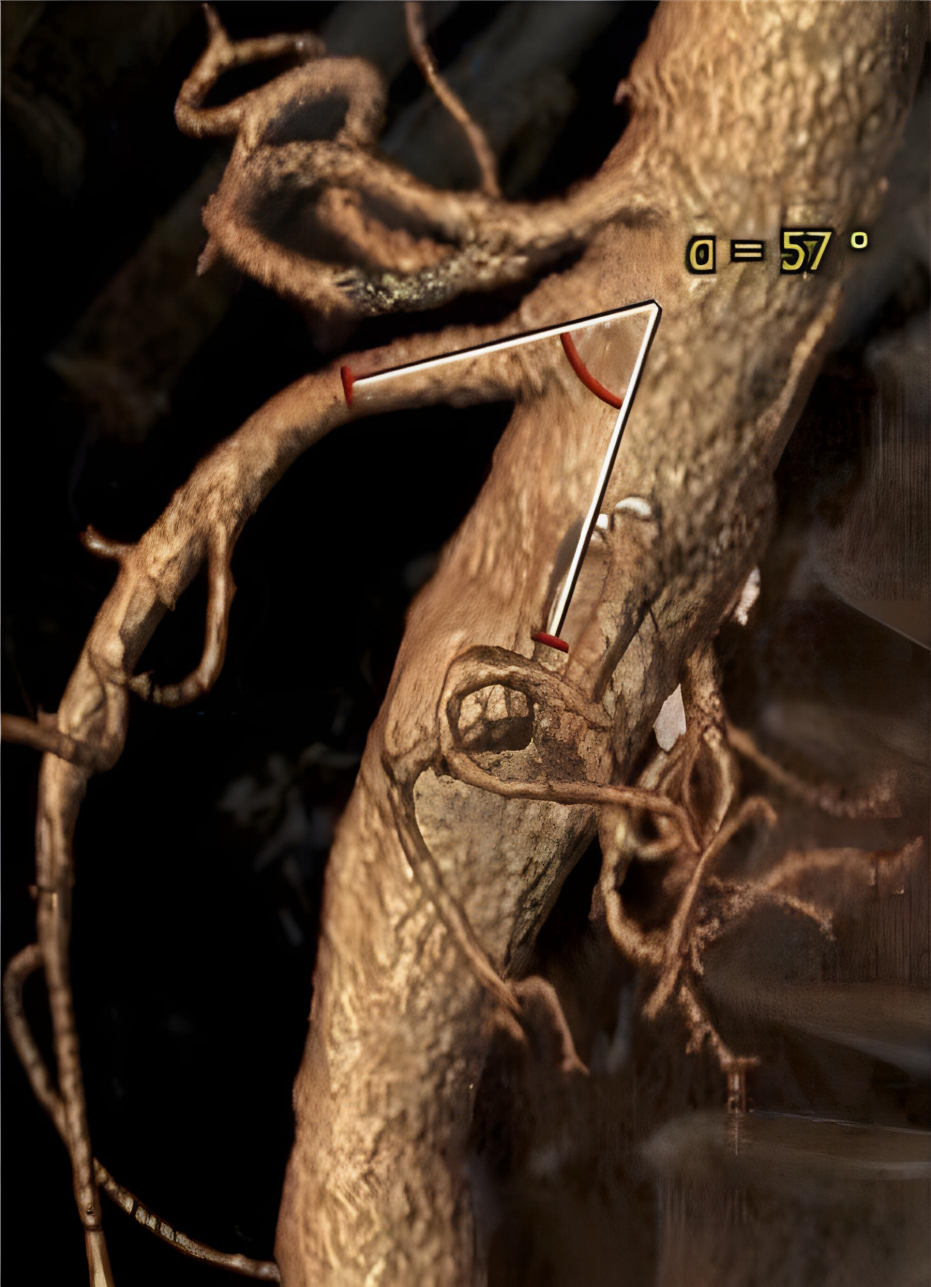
Volume rendering reconstruction of preoperative computed tomography angiography; angle between the longitudinal axis of the aorta and the main superior mesenteric artery trunk was evaluated by an electronic caliper provided by the 3-Mensio software.
